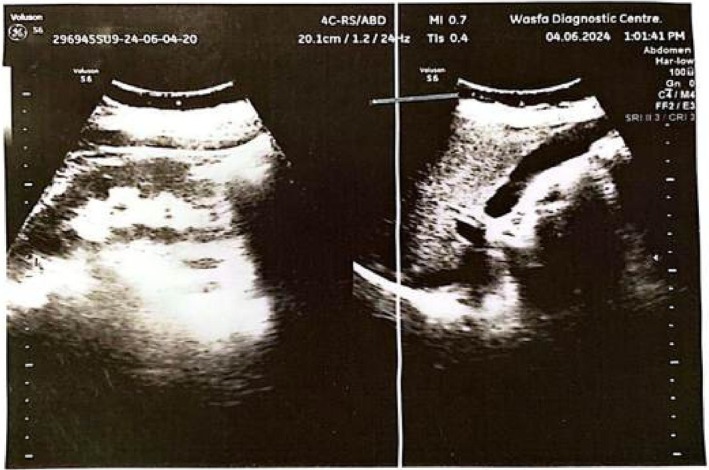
Ultrasound of Abdomen and Pelvis: Showed a calcified lesion at the bladder base with associated bladder wall thickening.
